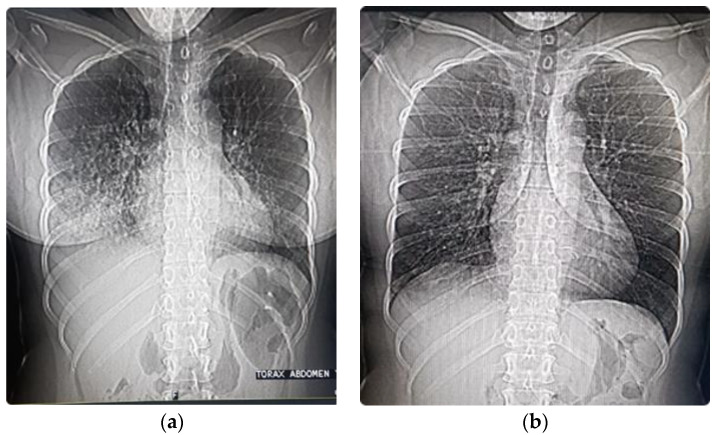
Thorax and abdominal X-rays performed during the CAT scan of a patient with HAPE at 3500 m. (a) Four days after arrival at high altitude. Note the distension of gases HAGE; (b) Two days later following the treatment described in our article [1].
